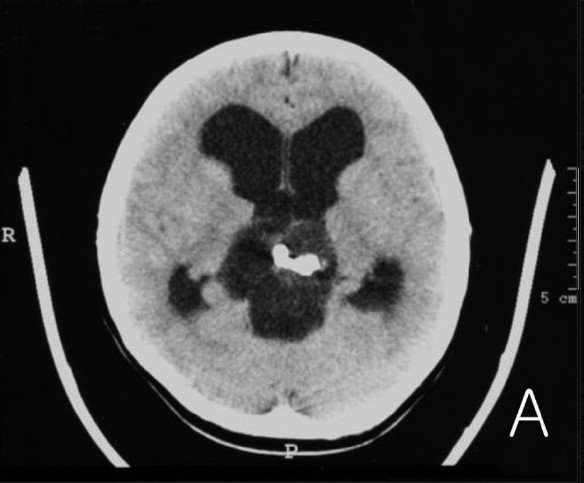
Preoperative brain computed tomography (CT) image showing a calcified cystic mass in the pineal gland (A)
